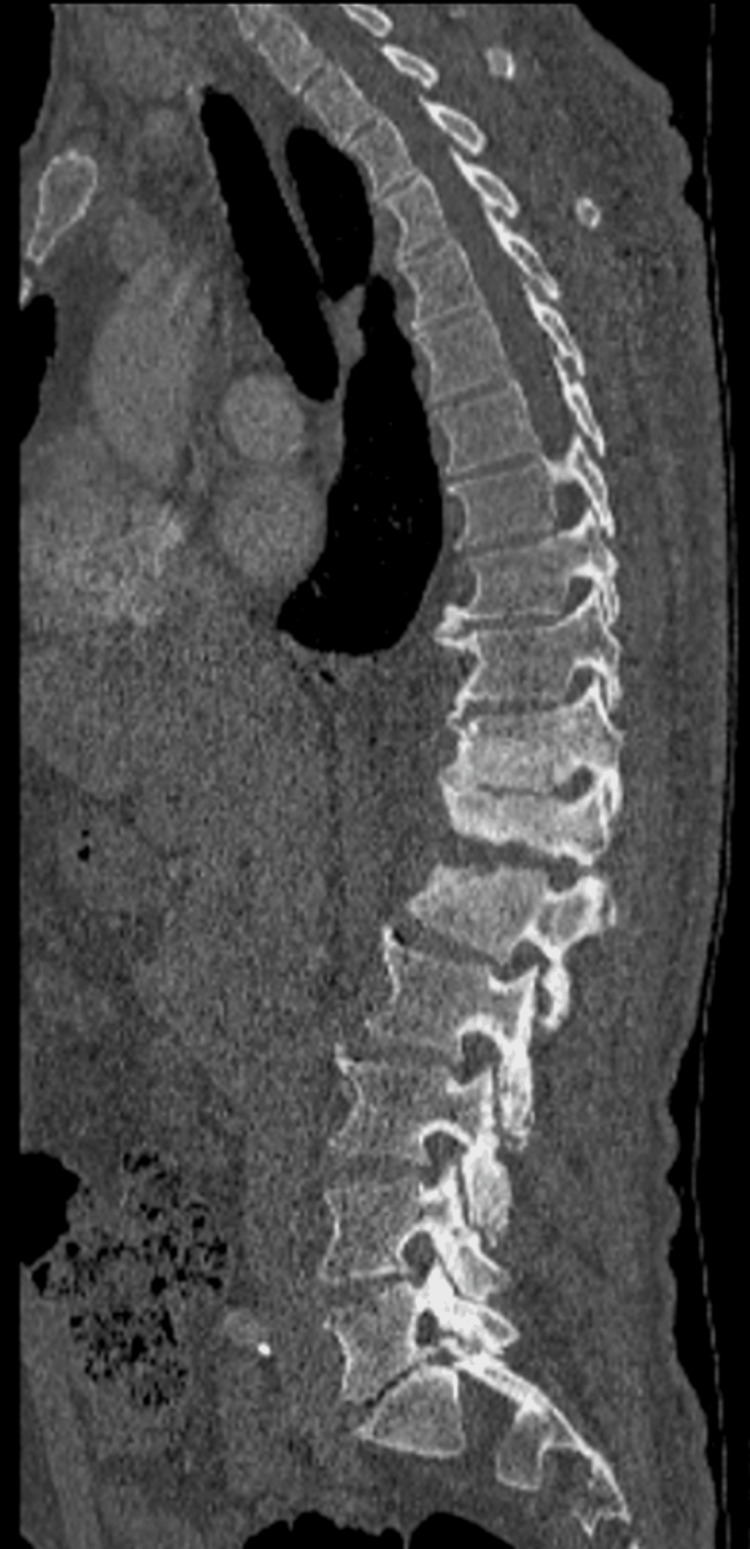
Non-contrasted lumbar spine CT scan. Lytic lesions at the level of T10, T11, T12, and L1. Loss of morphology in L1, T11, and T12. Loss of height T11-12. Adjacent collection in the psoas muscle on the left side and a mass that generates sclerosis in T11, T12, and L1.
